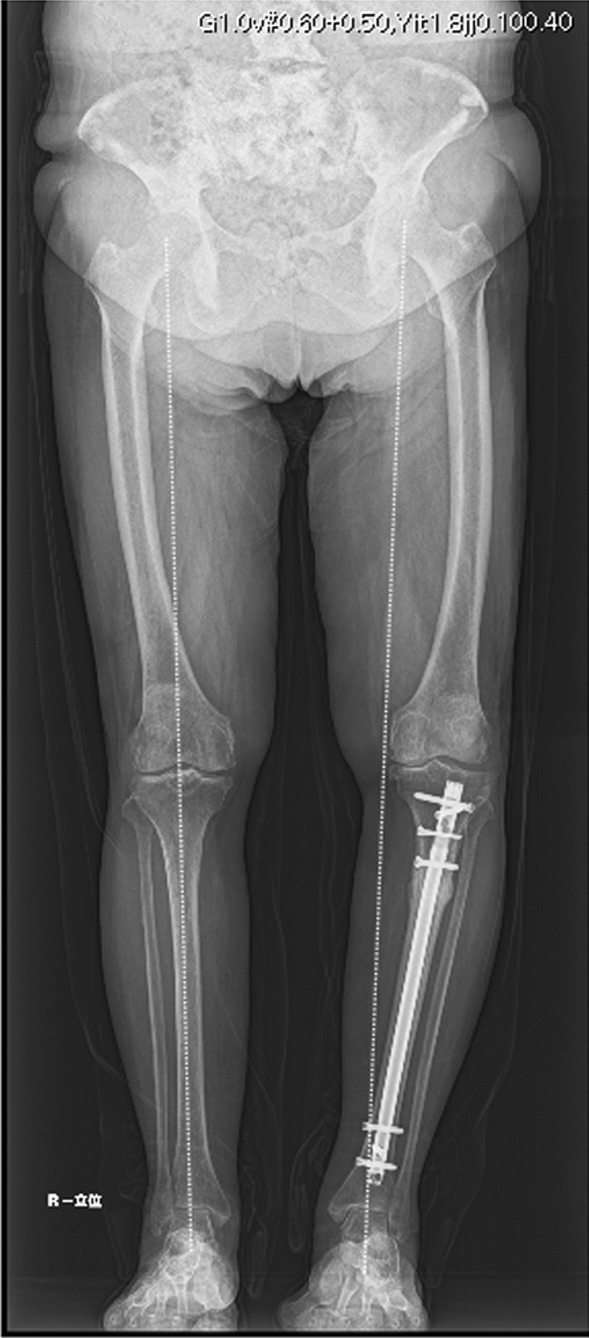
X-ray image of the lower limbs of the patient in the standing position, and the axis of lower extremity loading. The dotted lines indicate the bilateral Mikulicz’s lines of the lower extremity loading axis
